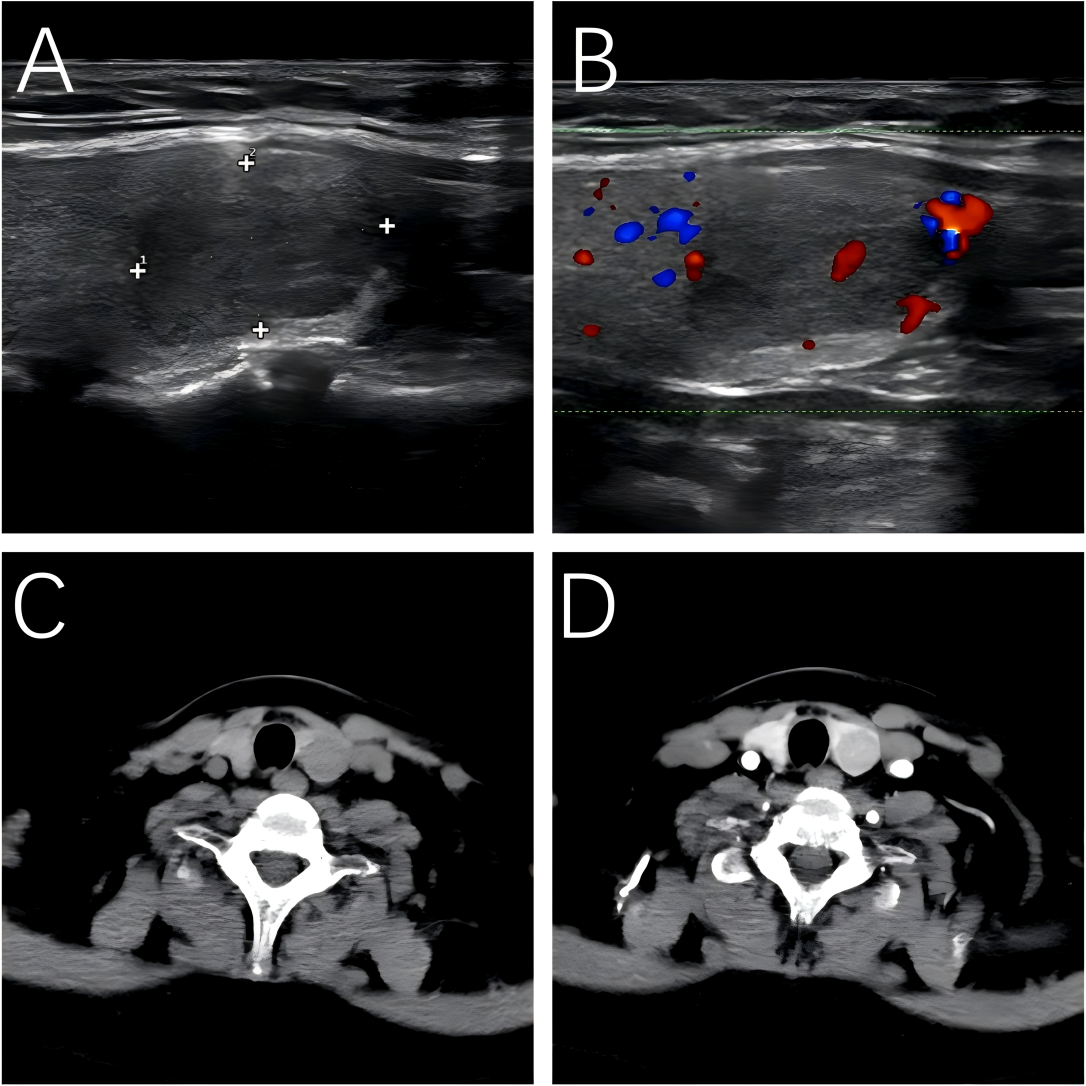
Ultrasound and CT-enhanced imaging of OA. (A) Ultrasound. (B) Color Doppler ultrasonography. (C) Axial view in CT scan. (D) Axial view in CT-enhanced scan.
